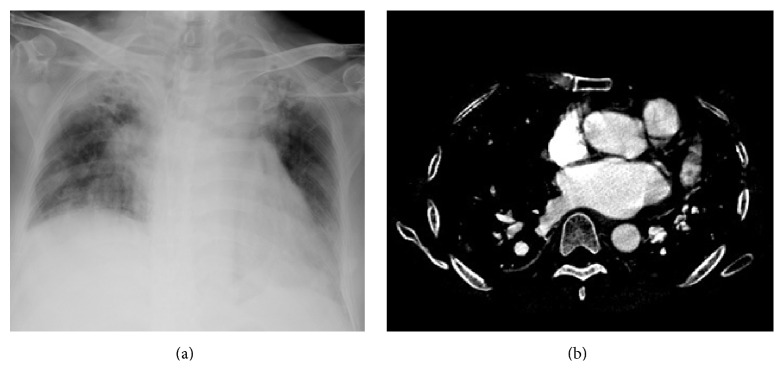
Central thrombus lesions. The patient is a 71-year-old woman with renal pelvis urothelial carcinoma who underwent chemotherapy. She felt dyspnea. A chest X-ray (a) shows bilateral lung hilar lesions. A CT scan (b) reveals bilateral thrombus/tumor located bilaterally within the superior pulmonary veins.
